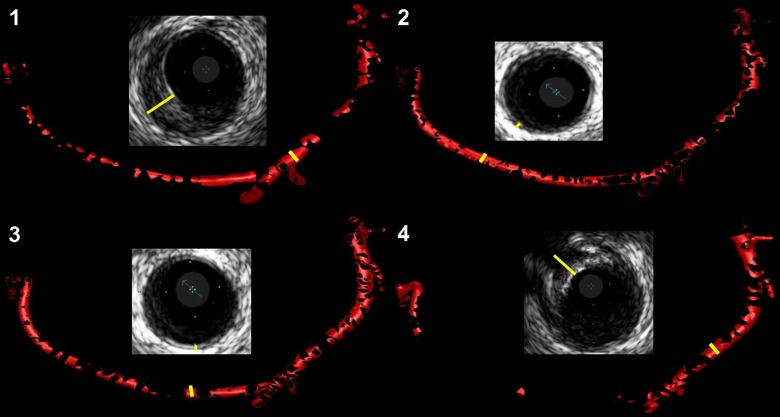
Vessel surface areas corresponding to different levels of endothelial shear stress (ESS). For each quartile of ESS a single model was reconstructed and used for correlation with intravascular ultrasound (IVUS). In quartile 1 (lowest ESS) and quartile 4 (highest ESS) a high prevalence of atherosclerotic plaque was observed whereas intermediate ESS (quartile 2 and 3) was mostly associated with no significant wall changes. The marked sites in the IVUS pictures correspond to marked areas on the surface models.
